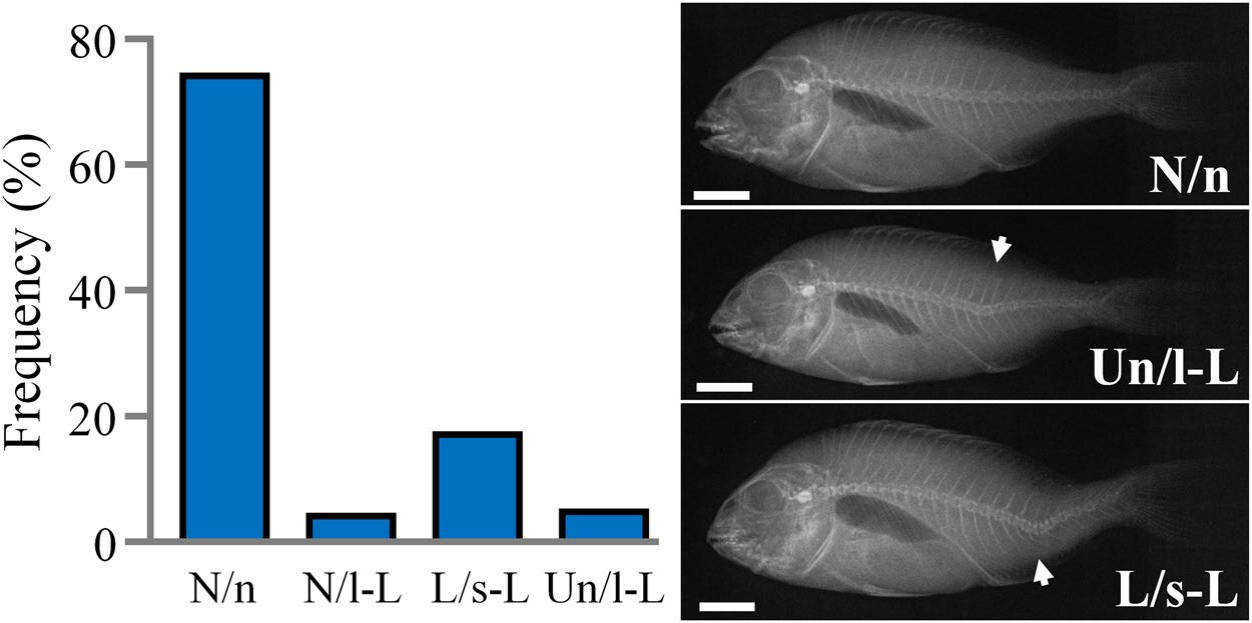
Frequency of the fish with normal (N), lordotic (L) and uncertain (Un) external morphology in the validation sample. Following the phenotypic categorization by means of external morphology, all individuals were examined in association with the radiographic appearance of the vertebral column (n, normal; l-L, lordosis of light severity; s-L, severe lordosis). Arrows indicated the lordosis center. Scale bars are equal to 1 cm.
